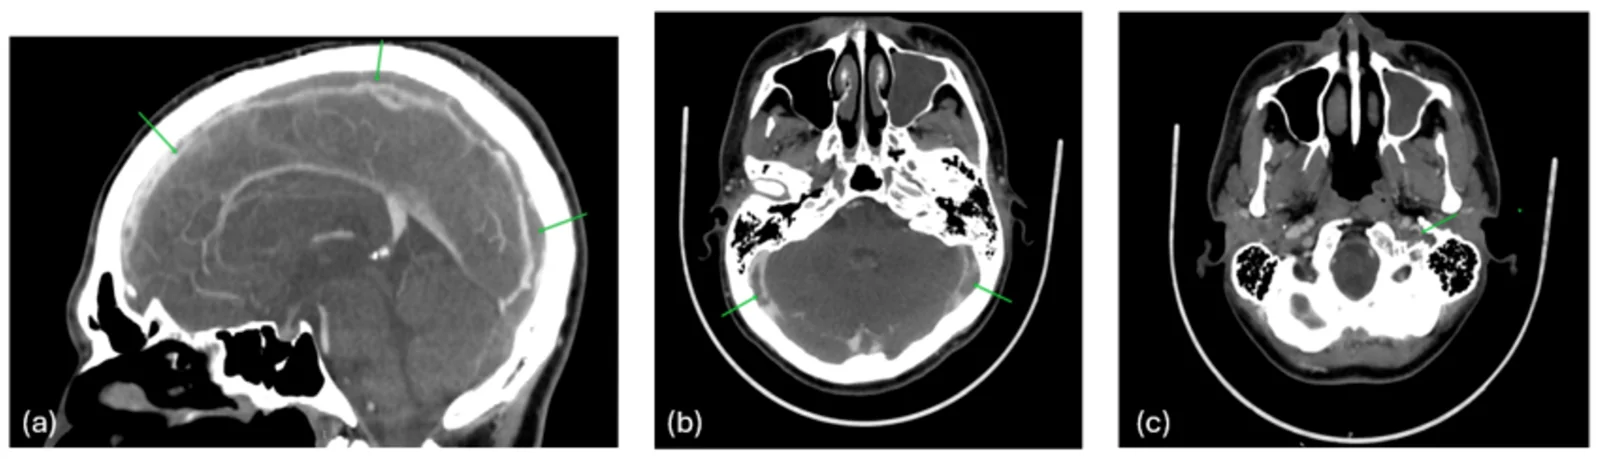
Cerebral Angio-CT revealed thrombosis (arrows) in the superior sagittal sinus (a), in the transverse sinuses (b) and in the left internal jugular vein (c).
